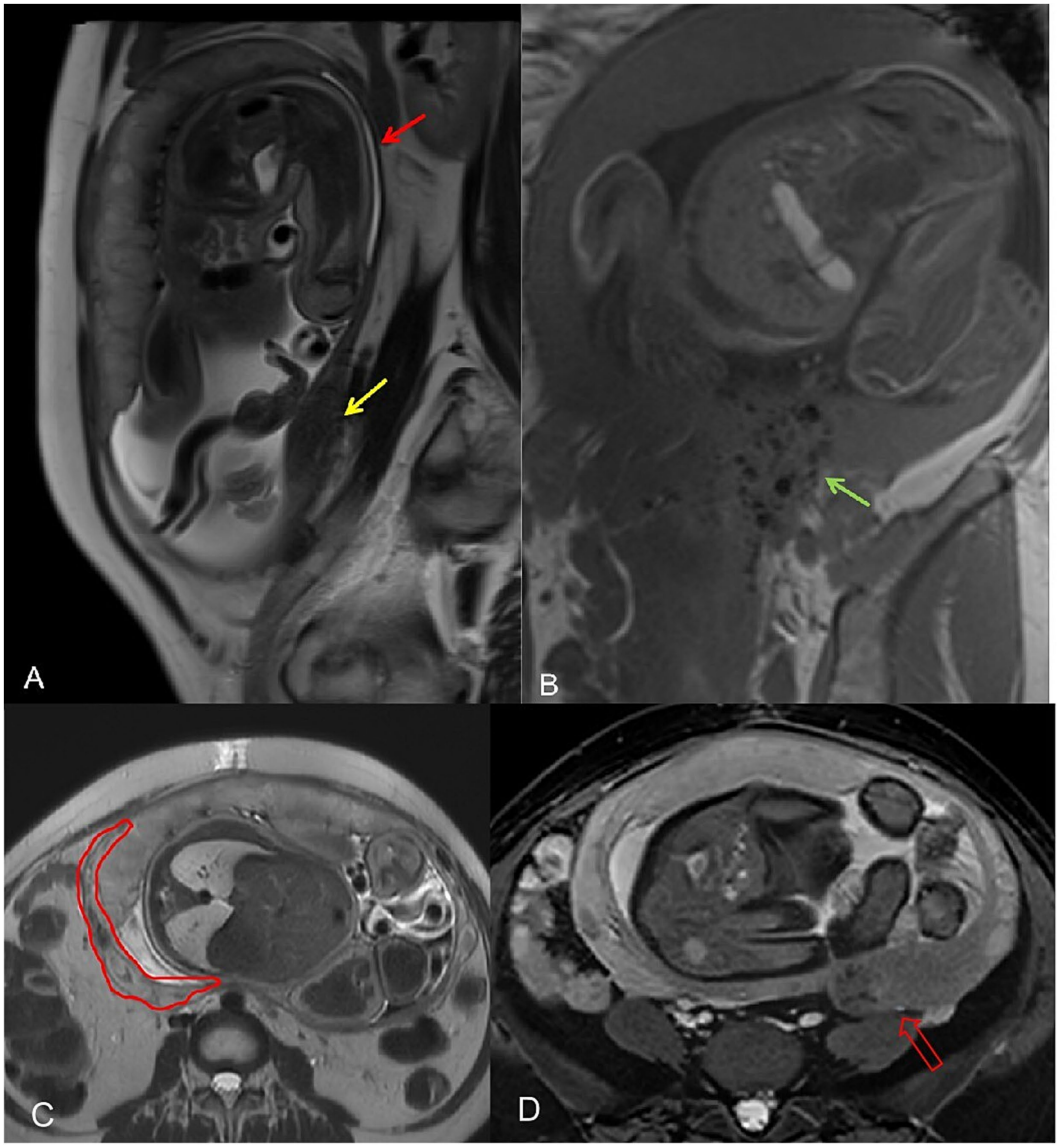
(A) The thinnest area of the myometrium in the posterior uterine walled arrow. A hypointense area is observed in the myometrium of the left posterior wall, considered to be an adenomyosis lesion (yellow arrow). (B) Postoperative speckled hypointense changes (green arrow). (C) The red outline indicates thickened intramural vessels, which were mistakenly interpreted as myometrial rupture on ultrasound. (D) The red hollow arrow points to the adenomyosis lesion area and the surrounding black speckled postoperative changes.
